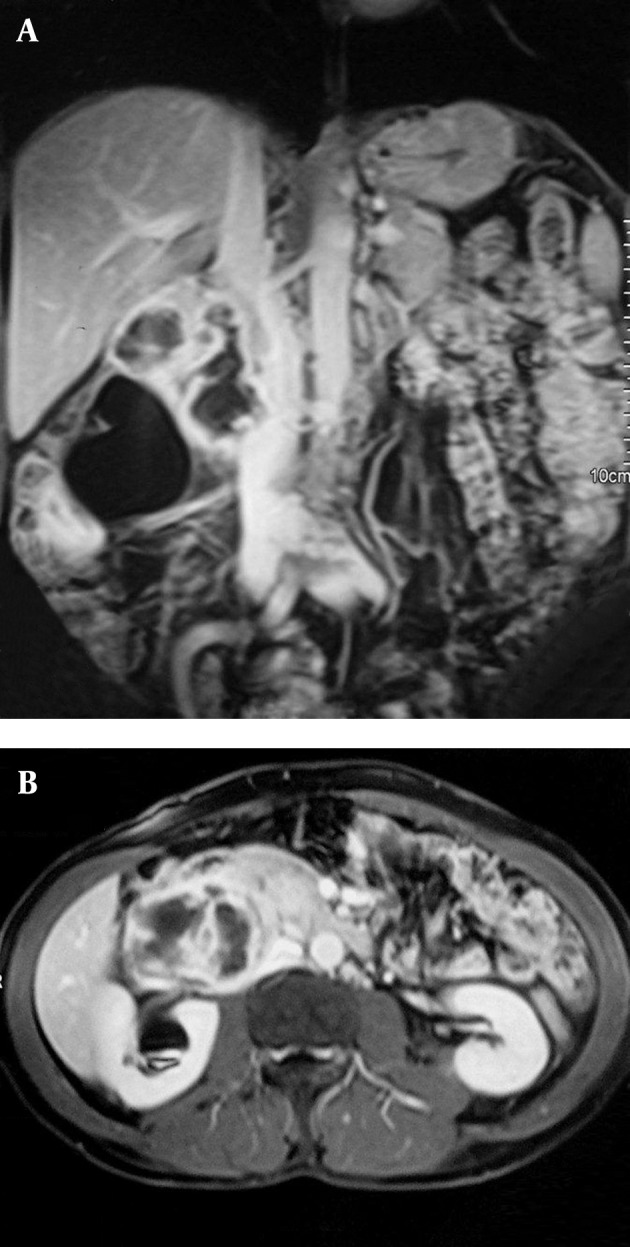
A. Coronal and B. Axial MRI following IV injection of contrast material shows a moderate level of heterogeneous contrast enhancement when compared to the surrounding muscle planes. The mass could not be separated from the inferior vena cava.
